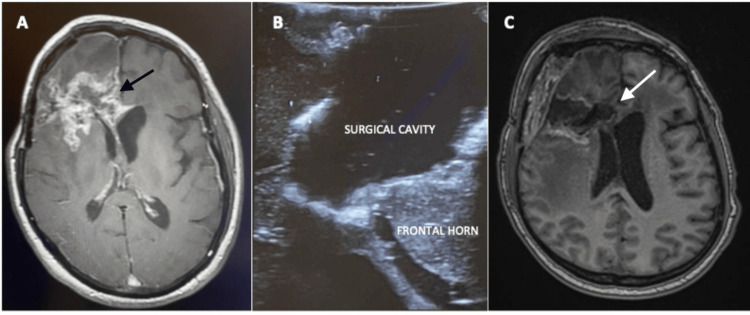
Resection of recurrent HGG (A) Preoperative T1+C image of a recurrent frontal HGG showing an irregular contrast-enhancing lesion in the right frontal lobe extending to the genu of the corpus callosum (black arrow). (B) iUS image after tumor resection. (C) Postoperative T1+C MR image showing GTR of the lesion and no visible residual contrast enhancement (white arrow). HGG: high-grade glioma; iUS: intraoperative ultrasound; GTR: gross total resection
